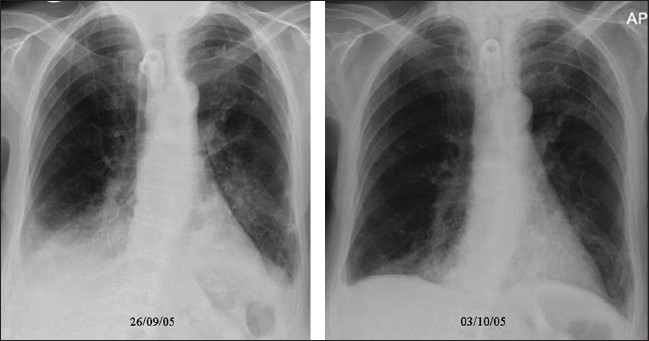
A series of AP radiographs on the same patient as in Figure 20 showing evolution of aspiration pneumonia at the right lung basewithin
